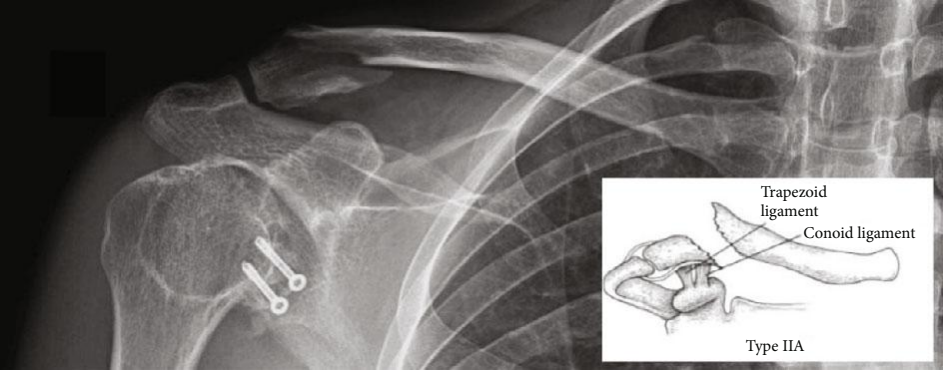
Standard radiograph of the right clavicle. It shows distal clavicle fracture classified as a Neer type IIA fracture pattern. Screws from the Latarjet procedure are in a good position without signs of loosening. The remnant of the coracoid process is also shown.
